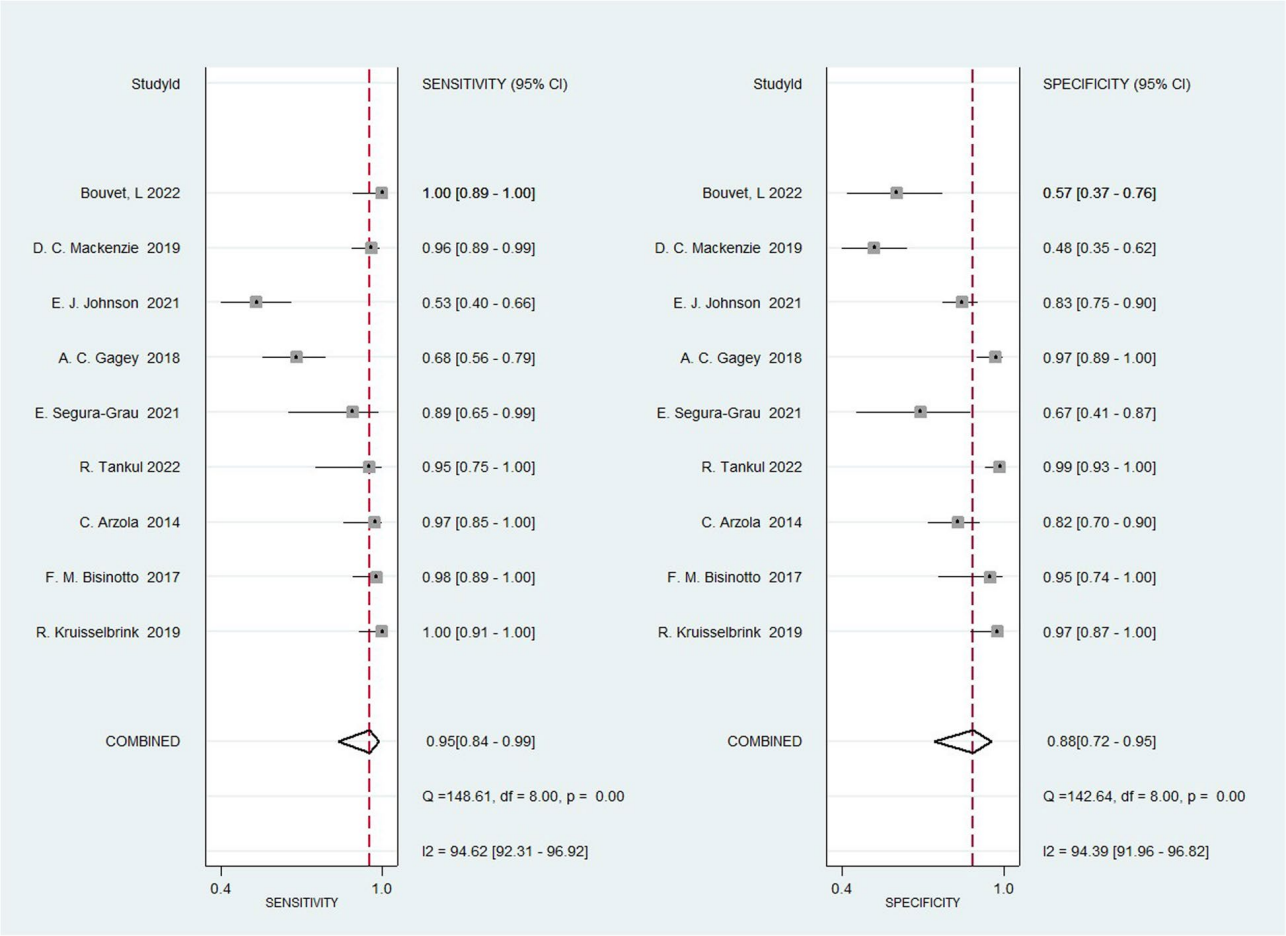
Combined forest plots for the sensitivity and specificity of ultrasound evaluation of gastric contents
